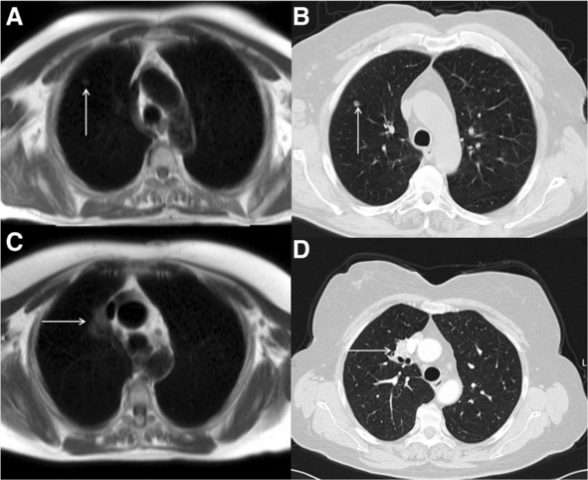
Axial black blood imaging (a) revealing an incidental 1.7 cm soft tissue density nodule in the right upper lobe (solid white arrows), which was subsequently confirmed with CT (b). Axial black blood imaging (c) demonstrating an incidental 3.1 cm soft tissue mass intimately related to the superior vena cava (solid white arrows), which was subsequently confirmed with CT (d)
